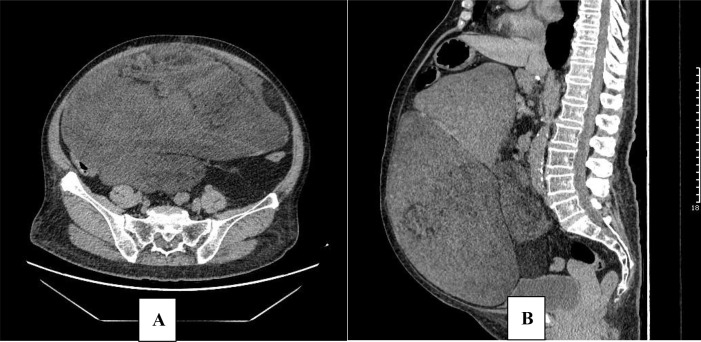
Abdominal CT. Abdominal CT obtained in October 2020 (cycle 2 of eribulin therapy). Stable disease (SD) confirmed by imaging data. Axial (A); Sagittal (B).
